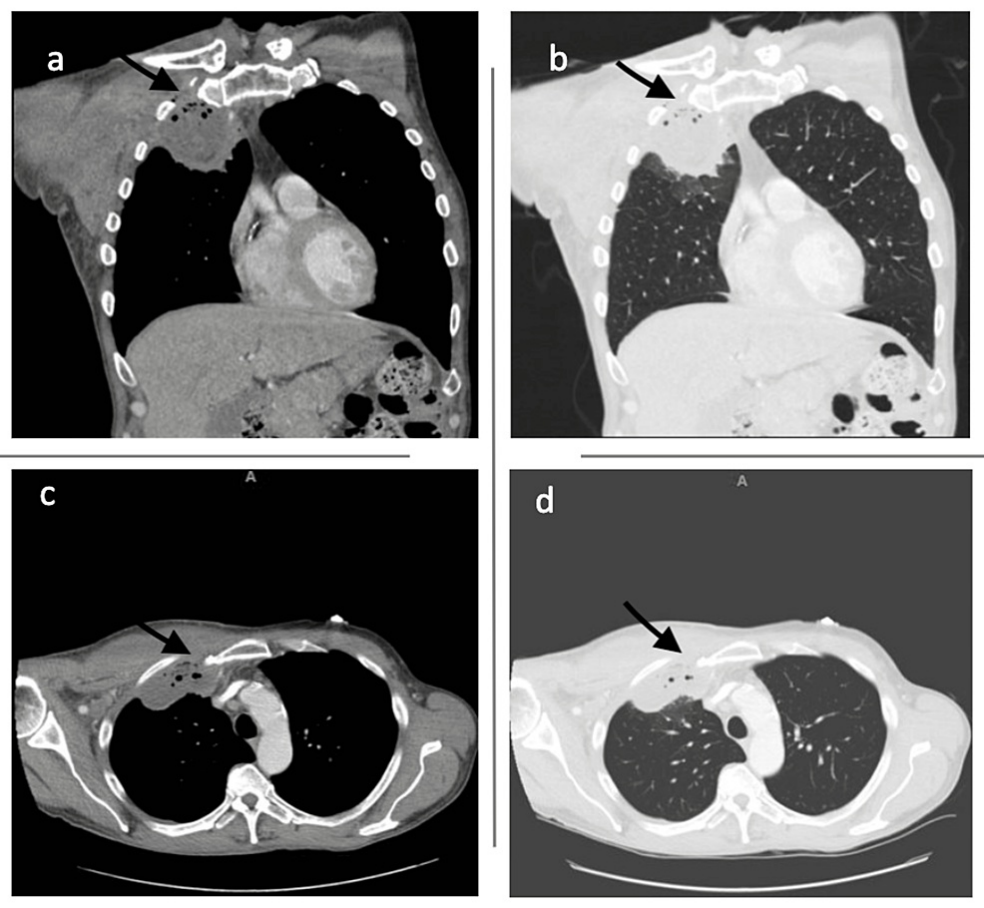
CT chest with IV contrast. Mass is present in the right upper lobe; (a) coronal view; (b) coronal view-lung window; (c) transverse view; (d) transverse view-lung window. CT: computed tomography, IV: intravenous
